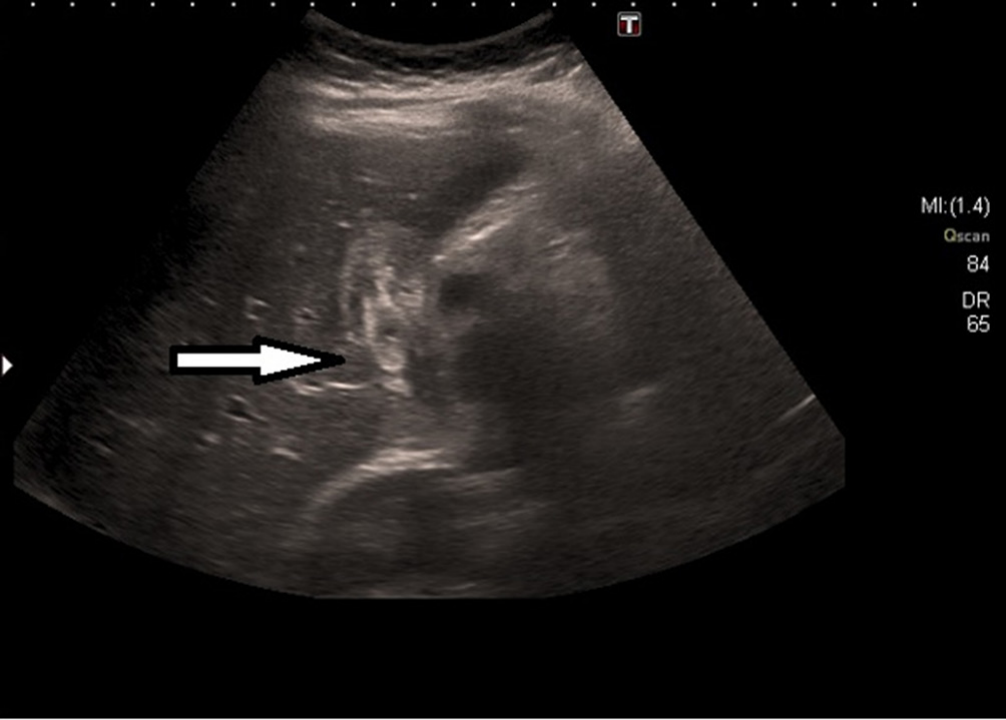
USS abdomen. On retrospective review, changes in keeping with biliary ascariasis were seen. Arrow denotes worm within an oedematous gallbladder with an almost "Bulls’ Eye" appearance. USS,ultrasound scan.
